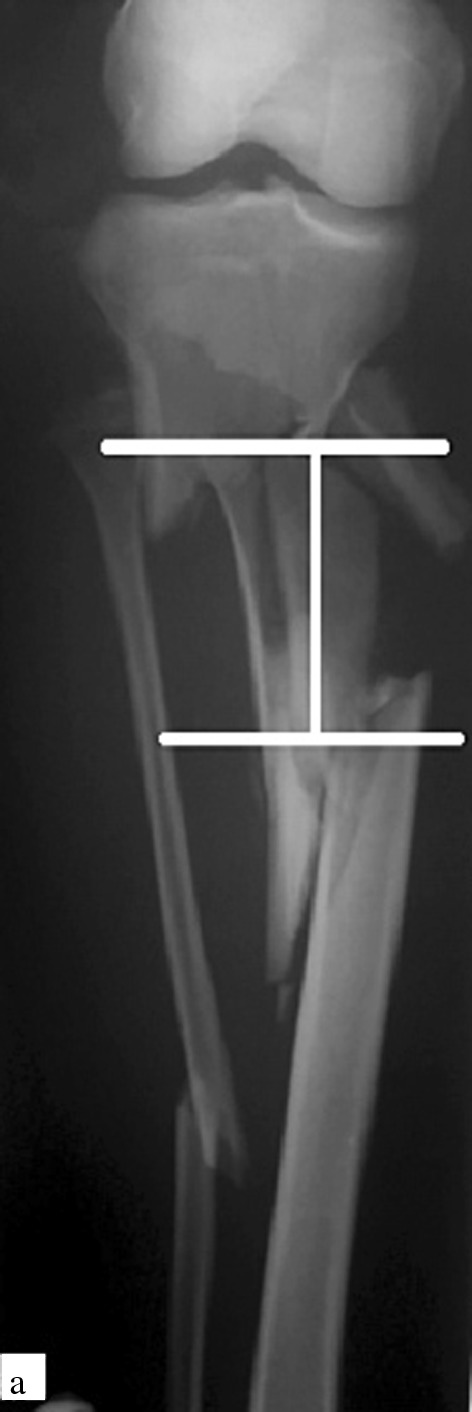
Antero-posterior radiograph showing comminuted fracture proximal tibia, 41-A3, with a segmental comminution gap of 64 mm
